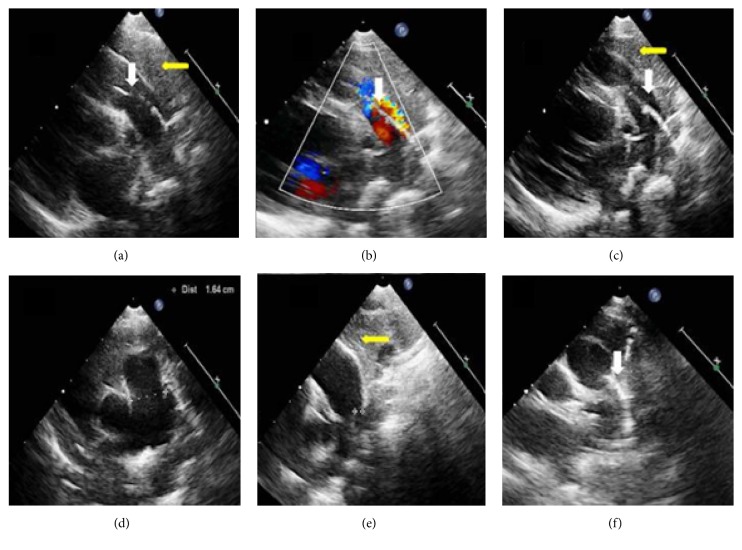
(a-c) Left high parasternal view. (d) Pulmonary artery long-axis view. (e, f) Near-suprasternal aortic short-axis view. (e) Location of the patent ductus arteriosus (white asterisks). A linear artifact is clearly seen (white arrowhead). The thymus gland is seen (yellow arrowhead).
